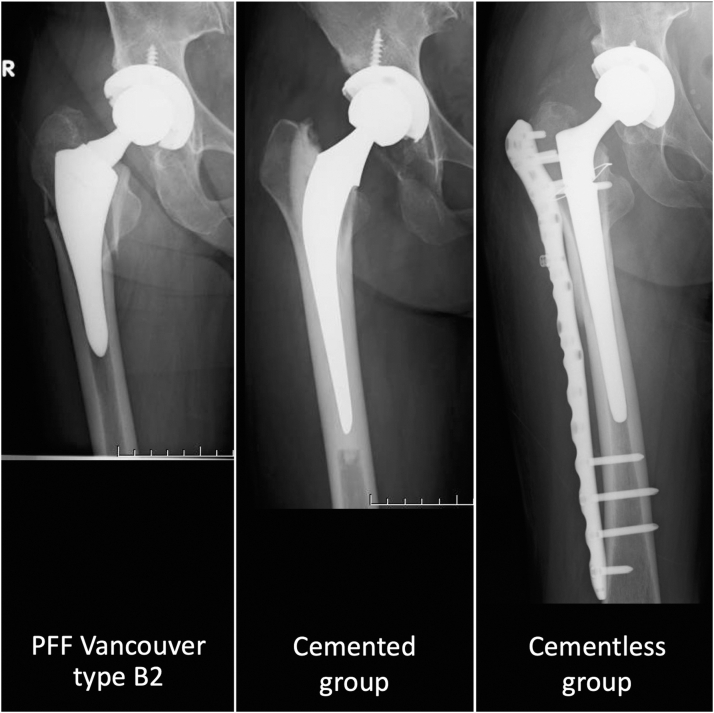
Representative radiographs of revision surgeries. A femoral fracture and sinking of the stem were observed and diagnosed as Vancouver type B2 periprosthetic femoral fracture (left picture). Representative radiographs of revision surgery with a cemented stem (center picture) and a cementless stem (right picture).
